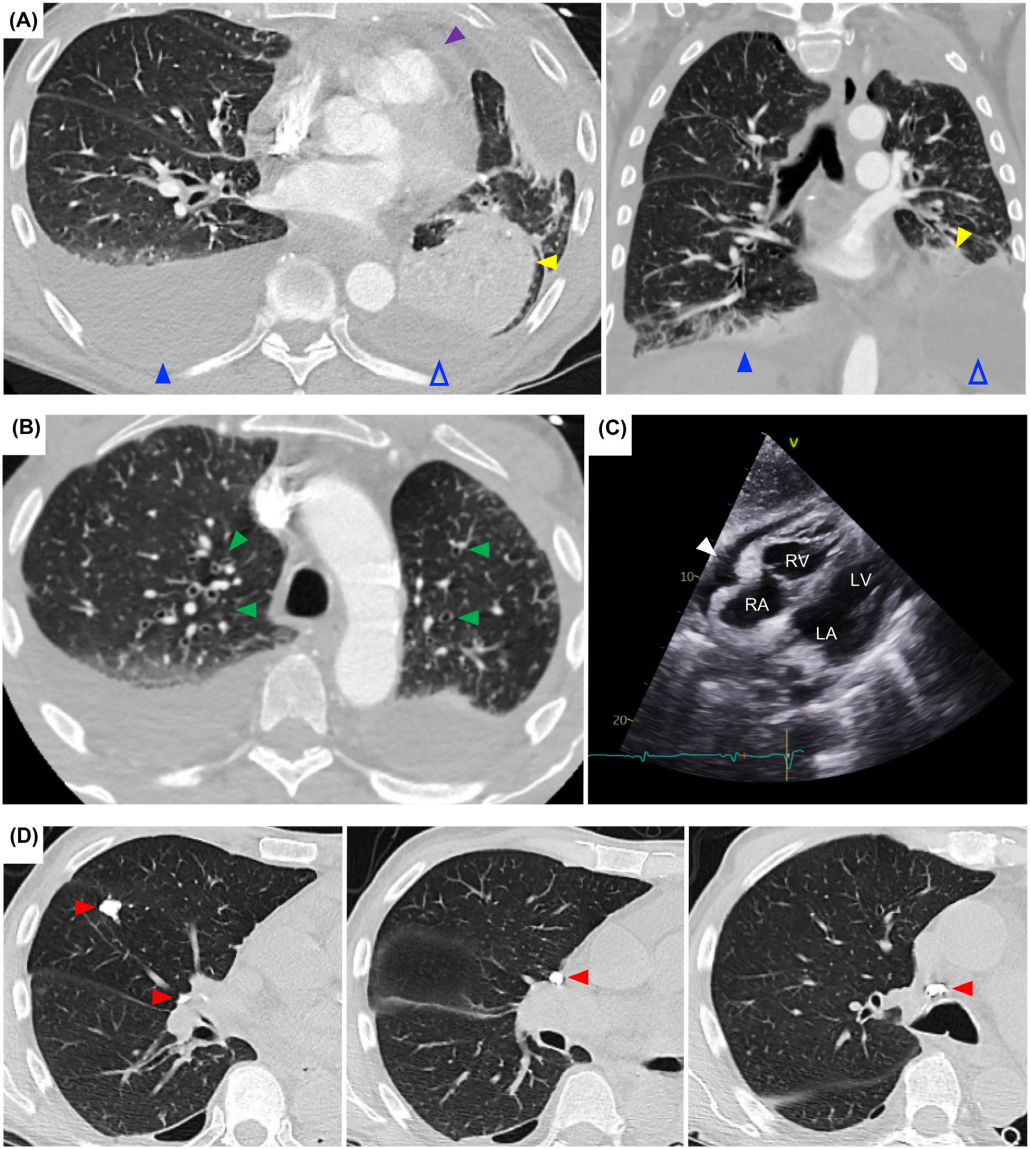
(A) Computed tomography angiography (CTA) showing moderate loculated left pleural effusion (open blue arrowheads), moderate free flowing right pleural effusion (filled blue arrowheads), rounded atelectasis in the left lower lobe (yellow arrowheads), and pericardial effusion (purple arrowhead). (B) CT without contrast showing bronchiectasis (green arrowheads). (C) Substernal view of transthoracic echocardiogram showing moderate pericardial effusion (white arrowhead) and right atrial wall inversion, concerning for tamponade. RA = right atria; RV = right ventricle; LA = left atria; LV = left ventricle (D) CT without contrast showing calcified granulomas (red arrowheads) in the right middle lobe and hilar/peritracheal lymph nodes
